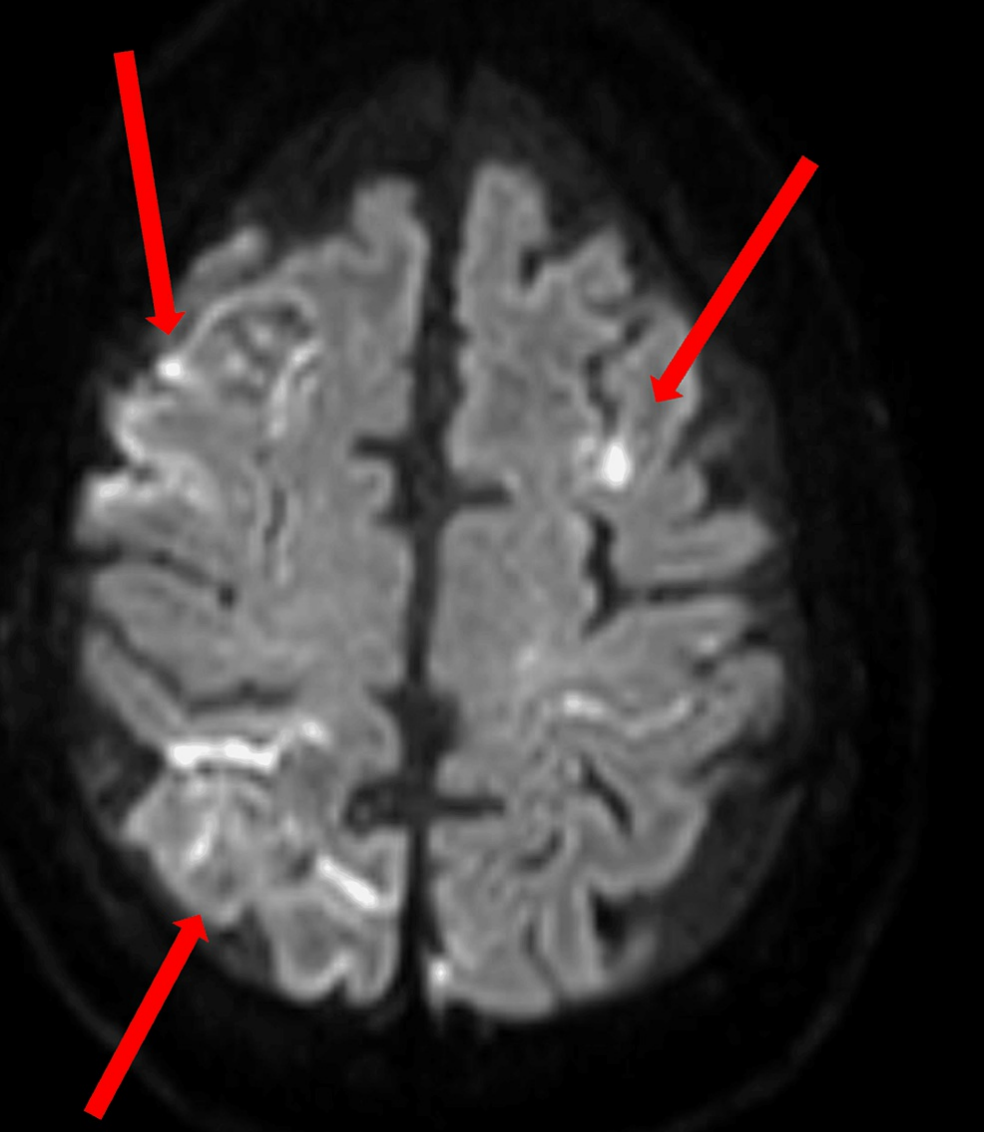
MRI diffusion-weighted imaging shows areas of increased signal, representing areas of acute subarachnoid blood and small cortical infarcts (arrows).
